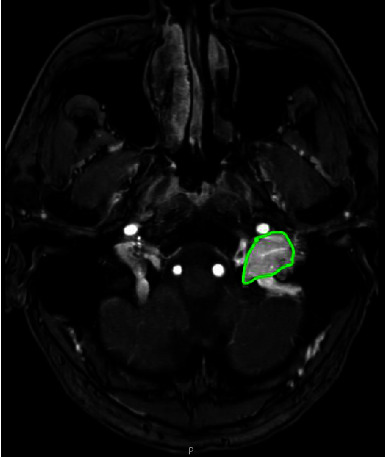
3D TOF with gadolinium MRI showing a jugulotympanic paraganglioma on the left side (green outline).
